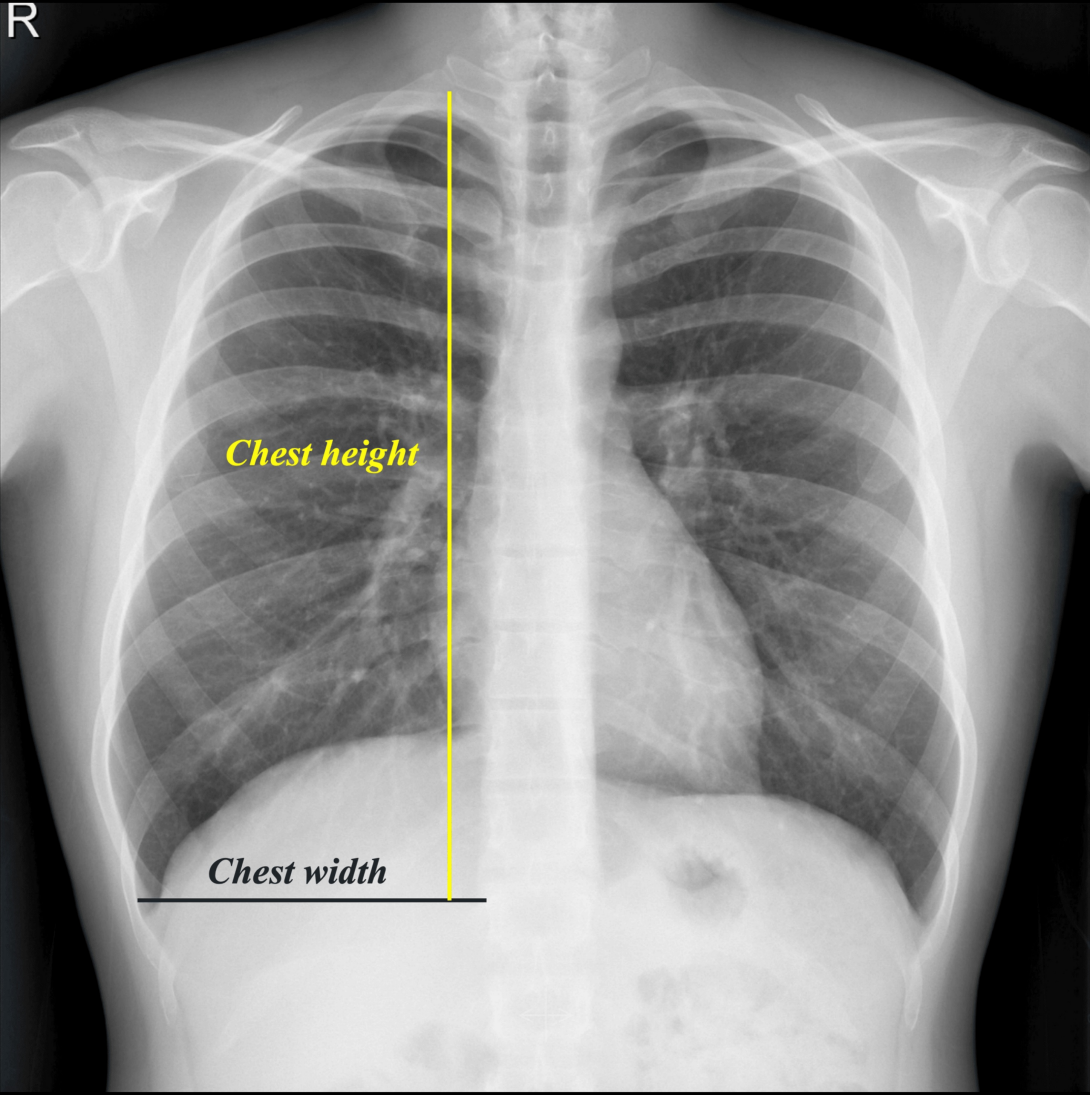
Measuring method of chest height and width from a PA CXR PA, posteroanterior; CXR, chest radiography
